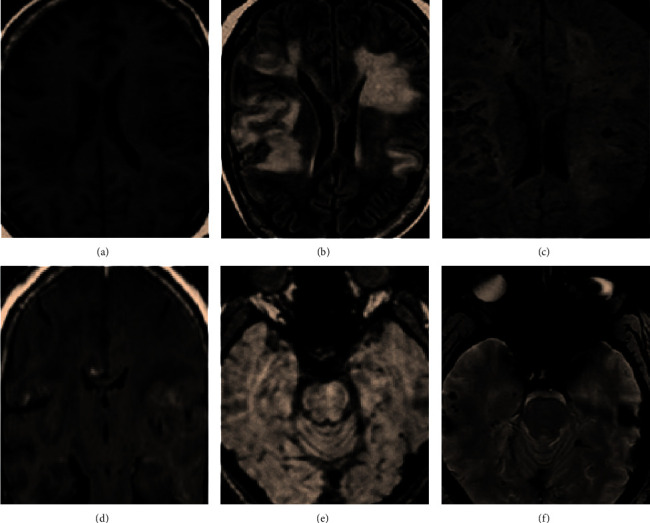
MI and SWI images of CMBs. (a–d) The image of T1WI, T2WI, T2-FLAIR, and DWI (*b* = 1000); these conventional MRI images showed no abnormality. (e) MI and (f) SWI image showed low-signal CMBs.
